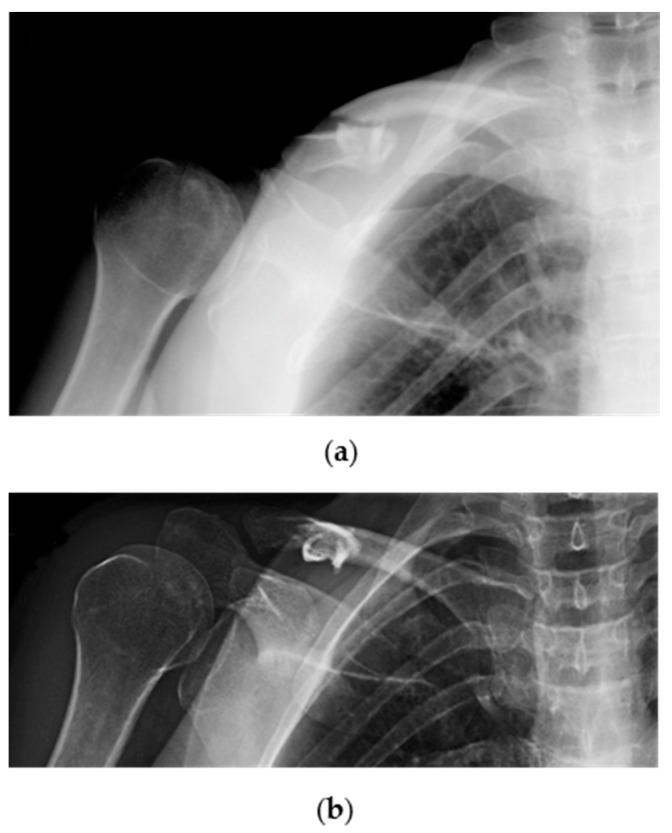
Radiographic images showing a traumatic displaced midshaft fracture of the right (dominant) clavicle in a 53-year-old female patient: (a) Cephalic tilt and; (b) anteroposterior X-ray views.
